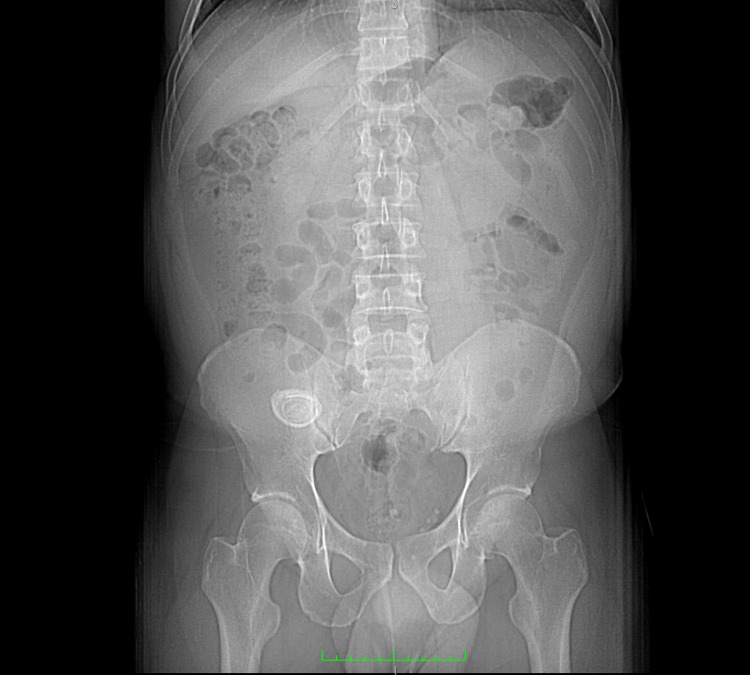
CT image showing an ovoid calcific density within the right iliac fossa, consistent with an appendicolith. CT: computed tomography
